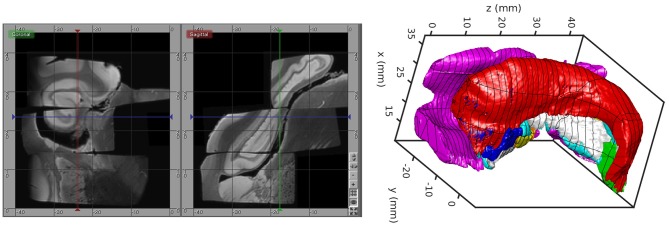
High-field MTL volume from MR. (Left) MR images of tissue blocks aligned in 3D. (Right) Surface rendering of manual segmentations with Mai Atlas coordinate system superimposed.
